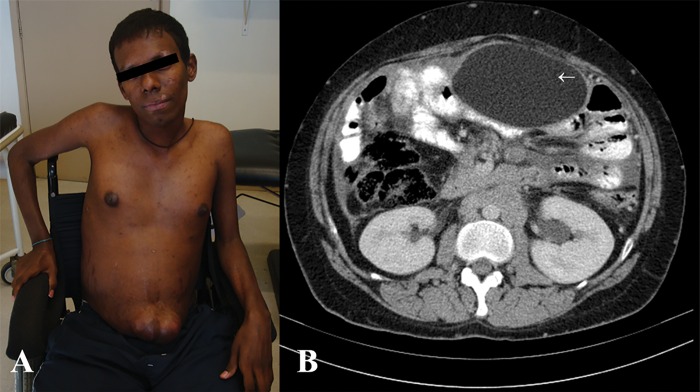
A. Patient’s abdominal bloating with a large umbilical hernia and scarring lesions in the face and trunk. B. Abdominal CT scan with oral and intravenous contrast reveals an 11.1 x 6.5 cm lesion with a density consistent with fat (-56 HU) in the anterior wall of the abdominal cavity (white arrow).
